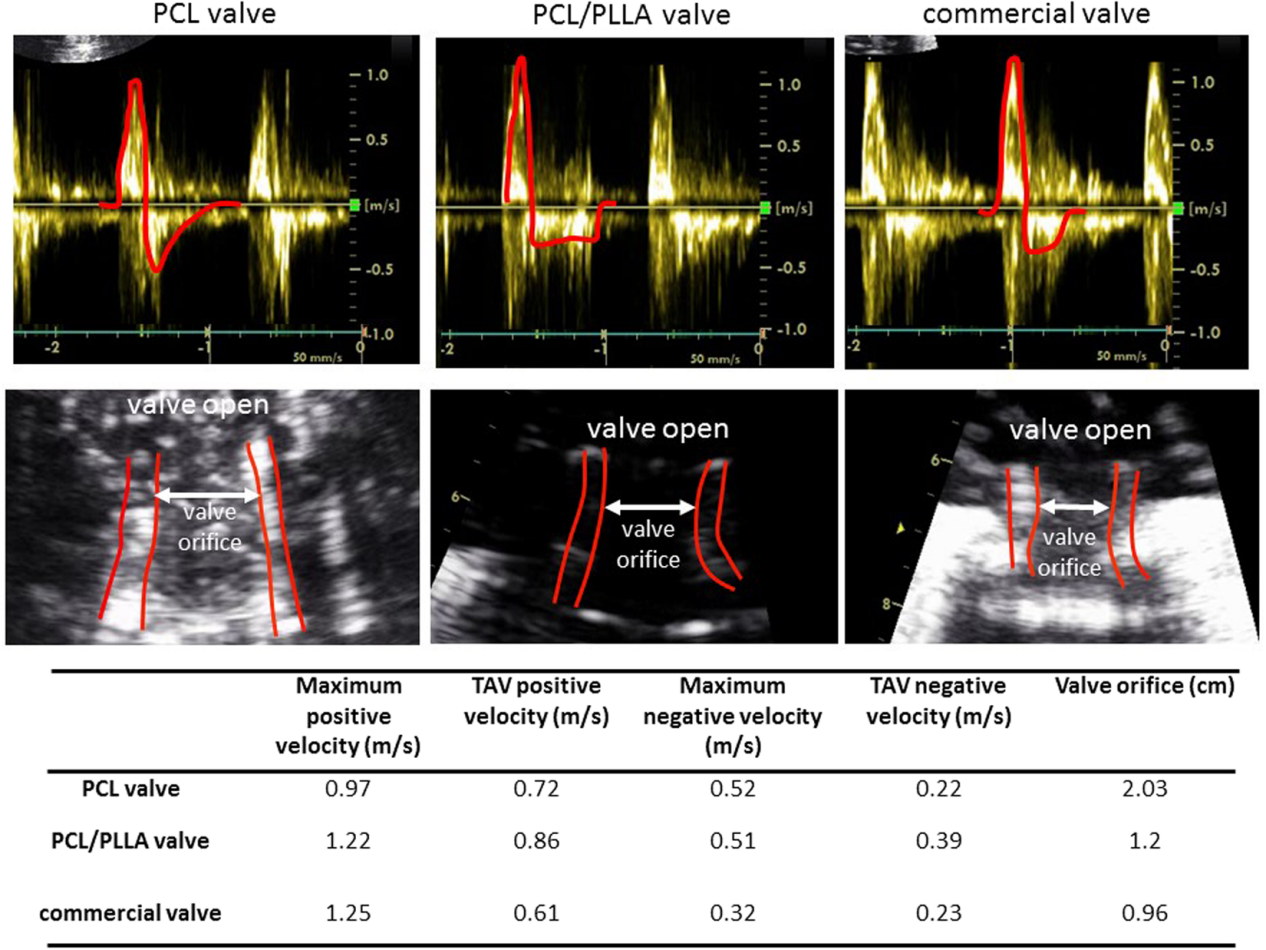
Doppler echo based experimental setup to test the fabricated heart valve compared to commercial valve. PCL valve results in maximum positive velocity value were lower than both the PCL: PLLA and the commercial valve, but time averaged value (TAV) of positive velocity for PCL valve was higher than the commercial valve and lower than the PCL: PLLA valve. Negative velocity values for PCL valve were lower than PCL: PLLA valve but higher than commercial valve showing regurgitation was more for the PCL valve compared to the commercial valve. PCL valve orifice size is more than both the commercial valve and the PCL: PLLA valve showing its superior opening characteristics compared to other two.
